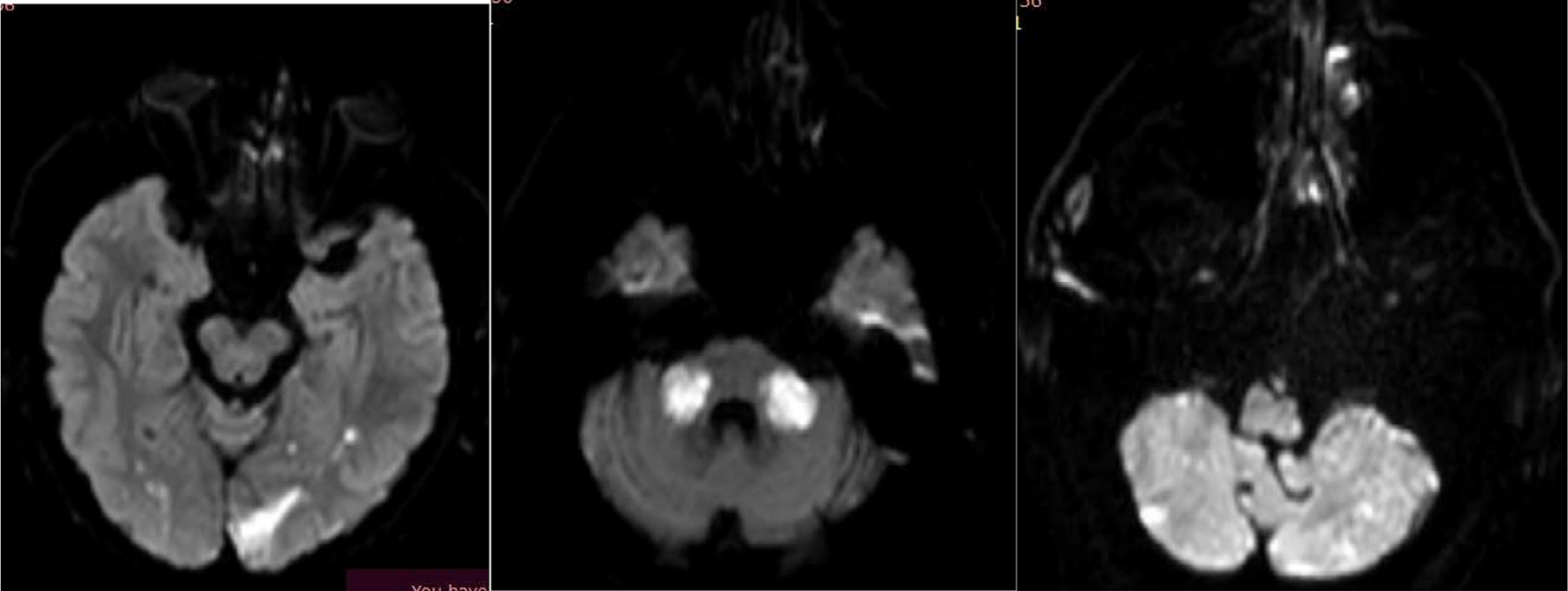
Demonstrate diffusion-weighted imaging (DWI) sequences revealing restricted water diffusion, indicative of ischemic lesions in the vertebrobasilar and posterior inferior cerebellar artery (PICA) regions.
